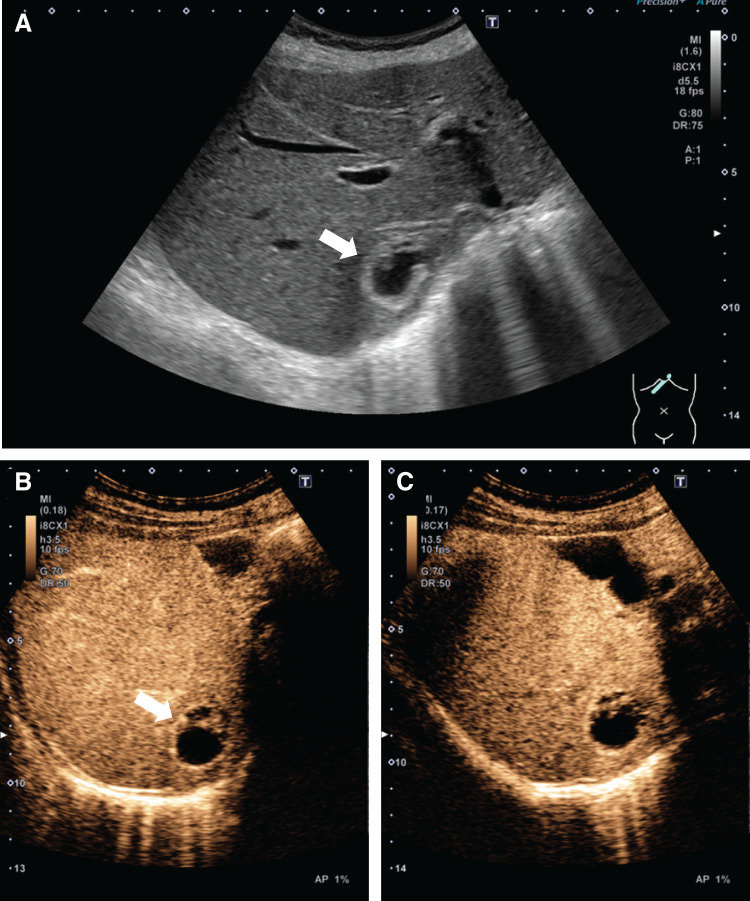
Abdominal ultrasonography findings before surgery. (A) B-mode ultrasonography showed homogeneous hypoechoic pattern with hyperechoic rim and septum-like structures (arrow). Contrast-enhanced ultrasonography using Sonazoid (GE Healthcare, Tokyo, Japan) demonstrated (B) enhancement only at the tumor periphery and septum-like structures (arrow), and (C) no clear defect in the Kupffer phase.
